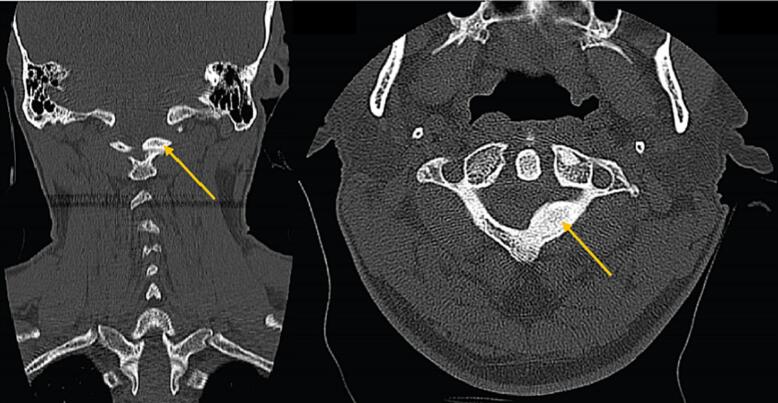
preoperative cervical computed tomography (CT) scan revealed the presence of a bony lesion on the left pedicle of the C1 vertebra that extends into the spinal canal.
